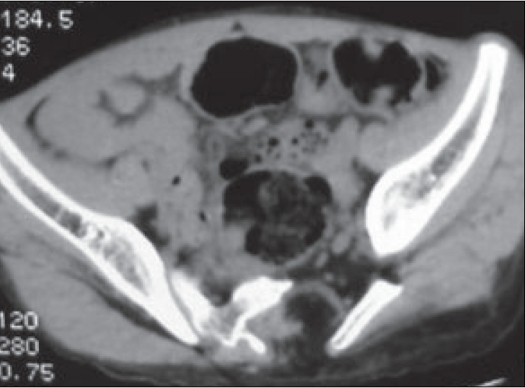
Axial CT showing absent left hemi sacrum with bony strutarising from the ilium
